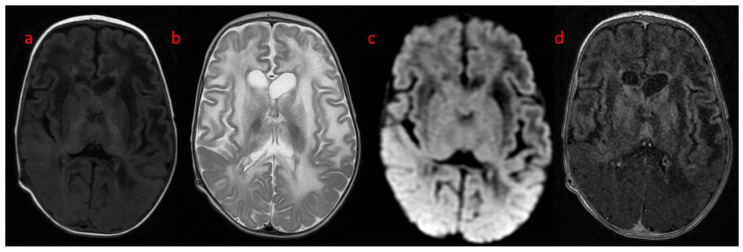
Brain MR at the 2nd follow-up (4th month of life): axial (a) T1, (b) T2, (c) DWI, (d) post-contrast T1: progression of signal abnormality, fluid collections at the frontal horns level.
